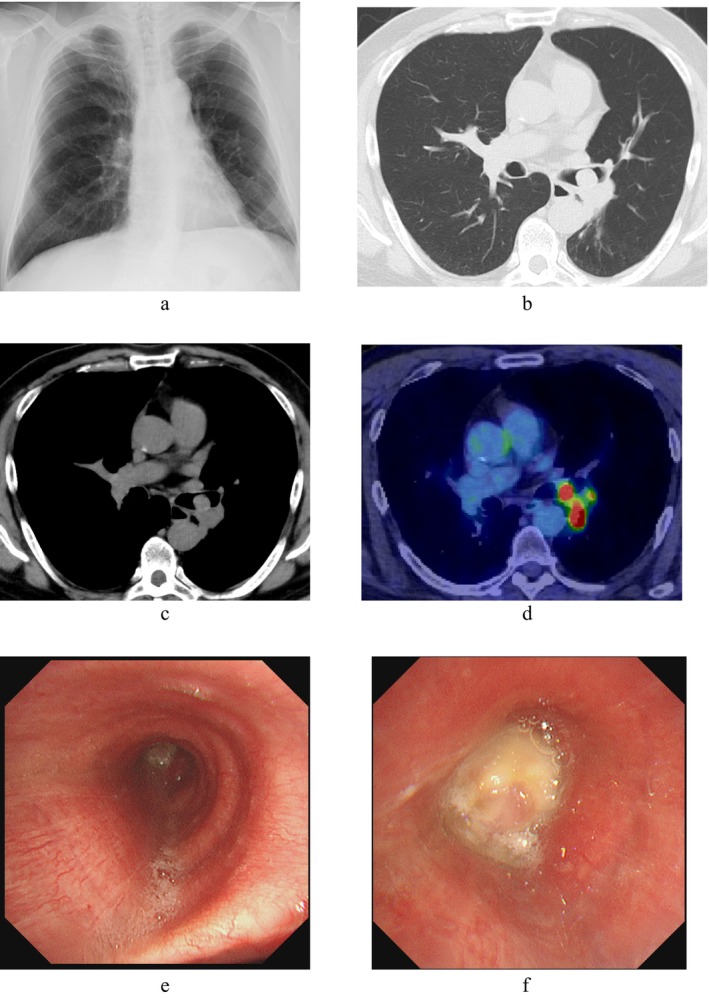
(a) Chest x‐ray, (b, c) computed tomography, and (d) ^18^F‐fluorodeoxyglucose (FDG)‐positron emission tomography/computed tomography (PET/CT) at first admission showed tumors in the left main bronchus and left hilar lymphadenopathy. (e) Bronchoscopy with standard observations from the carina revealed a tumor in front of the left second carina. (f) Bronchoscopy with standard observations showed a tumor obstructing the left main bronchus.
